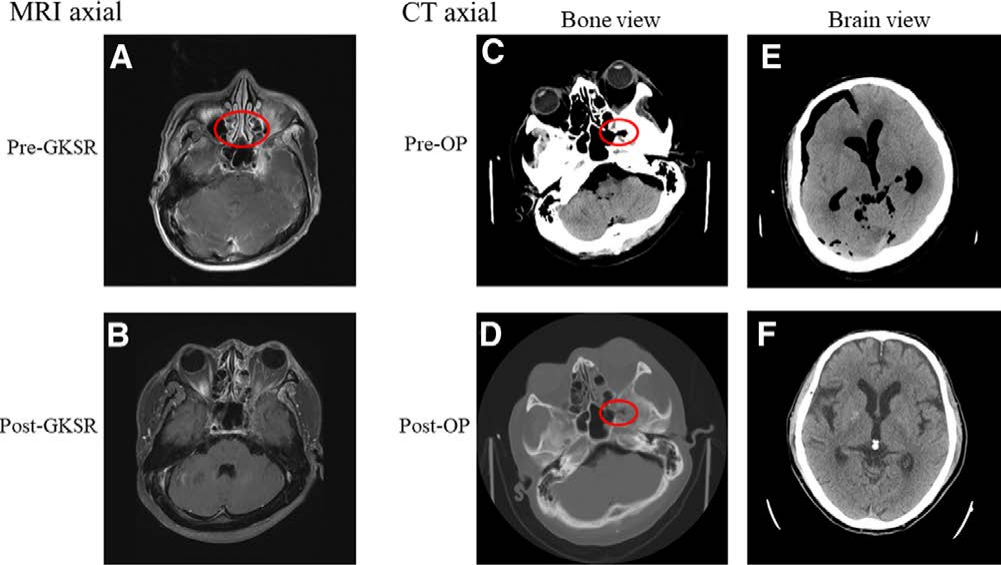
MRI and CT images at different times during the therapy. MRI image was taken at pre- and post-GKRS (A, B). Bone (C, D) and brain (E, F) view of CT pre- and after left frontotemporal (F–T) craniotomy. CT = computed tomography, GKRS = gamma knife stereotactic radiosurgery, MRI = magnetic resonance imaging.
